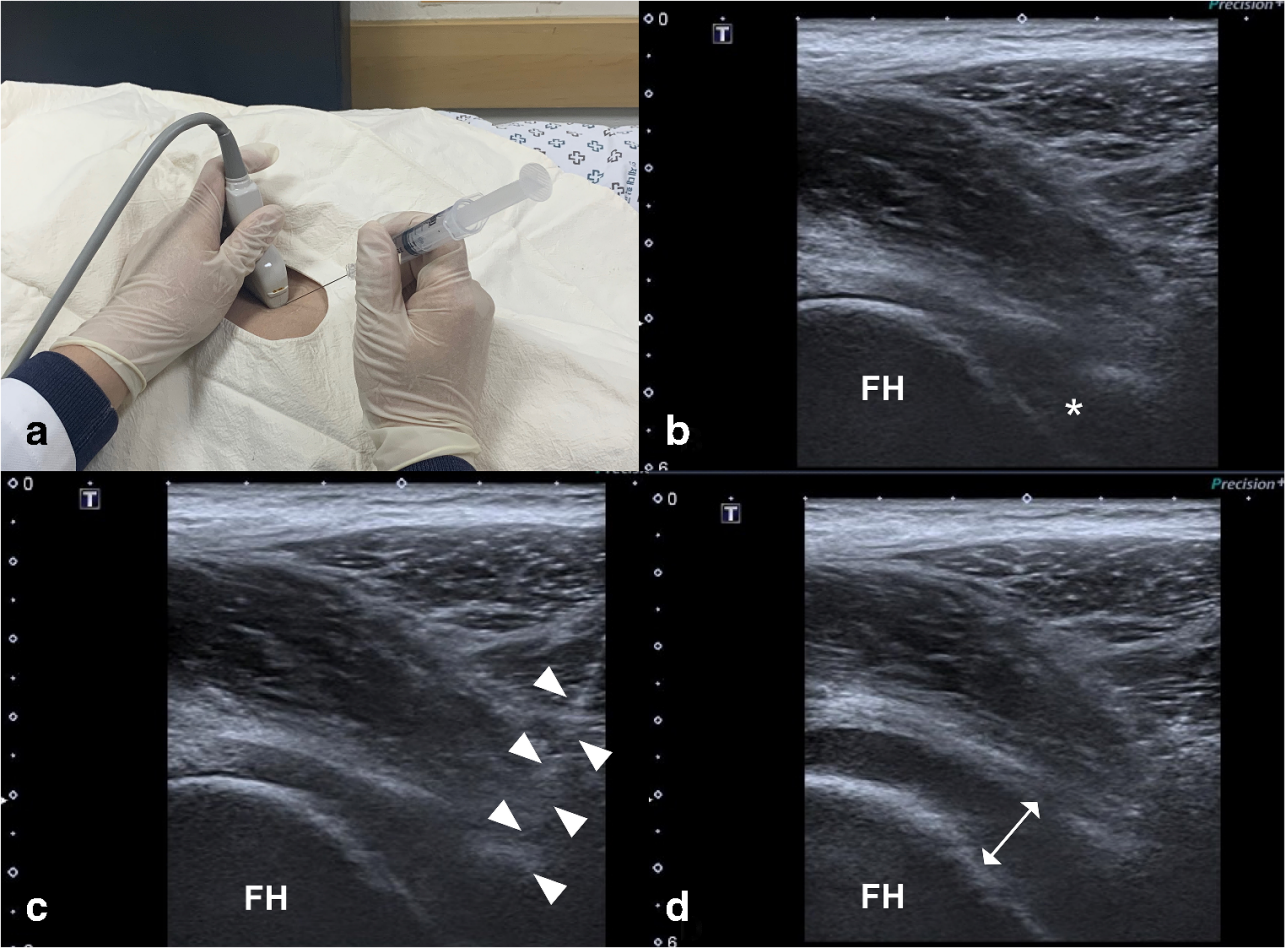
Images of the sagittal-oblique plane parallel to the femoral neck in a 47-year-old male patient in the supine position. Note the path of the needle to reach the joint cavity (white arrowheads). (A) Ultrasound-guided intra-articular injection was performed by placing the curvilinear transducer firmly over the area of the femoral head-neck junction in the long axis and slightly oblique view (anterolateral approach). (B) The anterior recess (*) of the hip joint over the femoral neck (normally about 4 to 6 mm thick) is targeted. (C) The needle is visualised as a hyperechoic line (arrow) and is targeted to the anterior synovial recess located at the neck of the femur. Two millilitres of fluid was injected to confirm the location of the needle tip. (D) After ultrasound-guided injection, maximum tolerable distension was achieved without rupturing the capsule (double arrow). FH, femoral head; AR, anterior recess of hip joint
